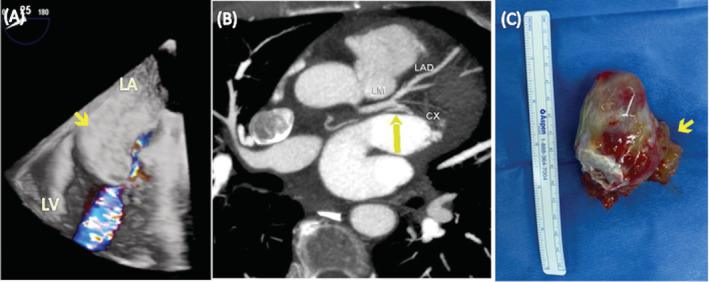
A, Narrow: 3D transesophageal echocardiography reveal the mass had prolapsed into the left ventricular inflow tract without adhering to the mitral valve. B, Computed tomography revealed a large mass attached to the interatrial septum with a broad stalk and an afferent artery (arrowhead) dependent on the circumflex coronary artery. C, Mass measuring 75 × 60 × 60 mm was removed during surgery with his pedicle (arrow)
